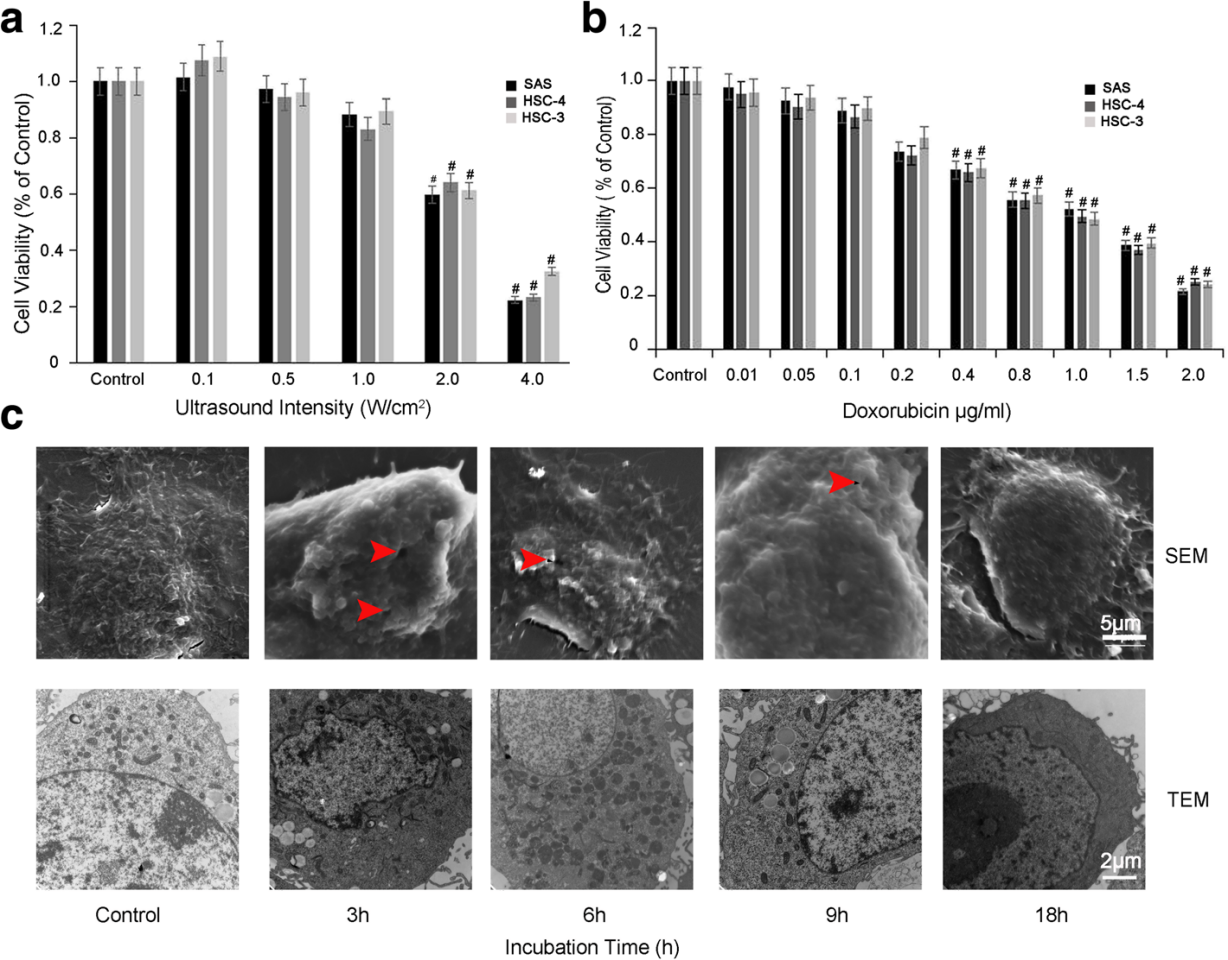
Cytotoxic effects of LIUS and DOX on OSCC cells. Cytotoxic effects were measured by MTT assay after exposure to different ultrasound intensities (a) and different DOX dosage (b) for 24 h. Data are expressed as means ± SD of three independent experiments. c, The morphological and ultra-structural changes of SAS cancer cells were detected by SEM (*upper panel*) and TEM *(lower panel*), at different incubation times after 1 W/cm^2^ ultrasound exposure
